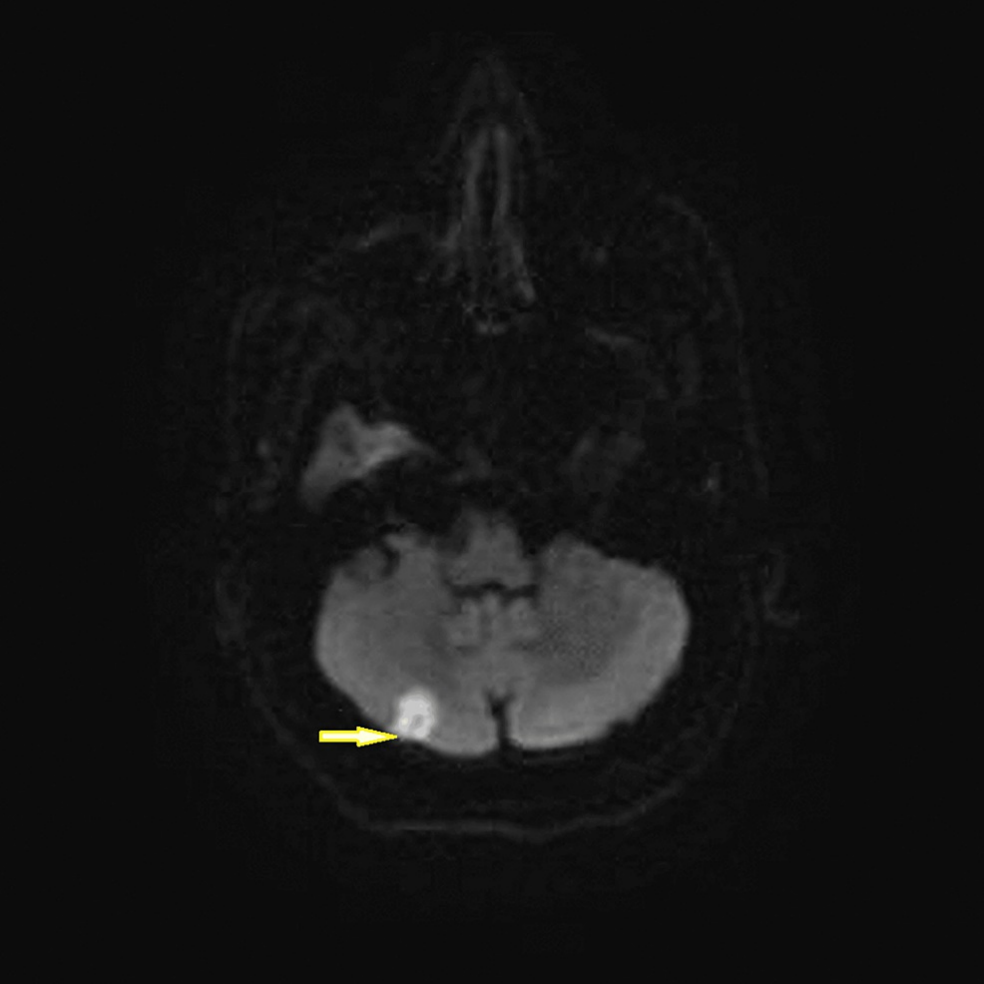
MRI brain without IV contrast with new right cerebellar infarct (axial T2 image, arrow).
